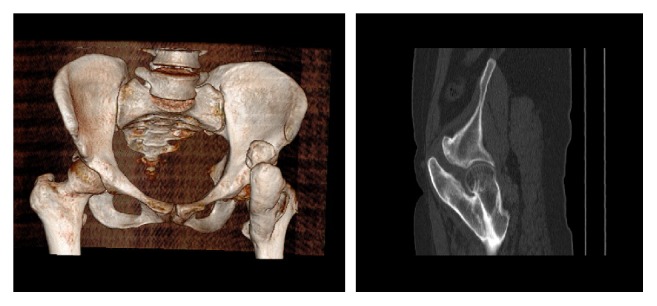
Preoperative radiographic evaluation with 3D and sagittal view of CT.
